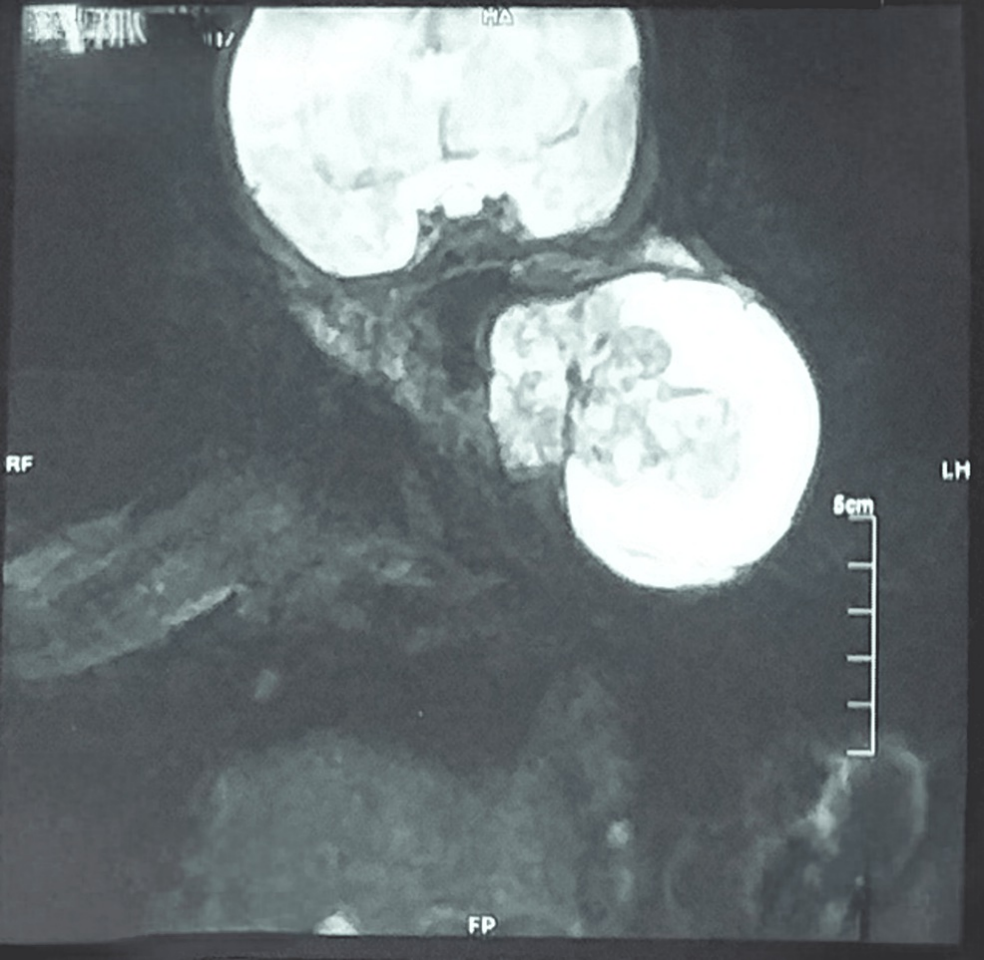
T1 weighted MRI coronal view after contrast enhancement showing complex solid and cystic lesion in left submandibular region, extending over left lateral aspect of neck.
